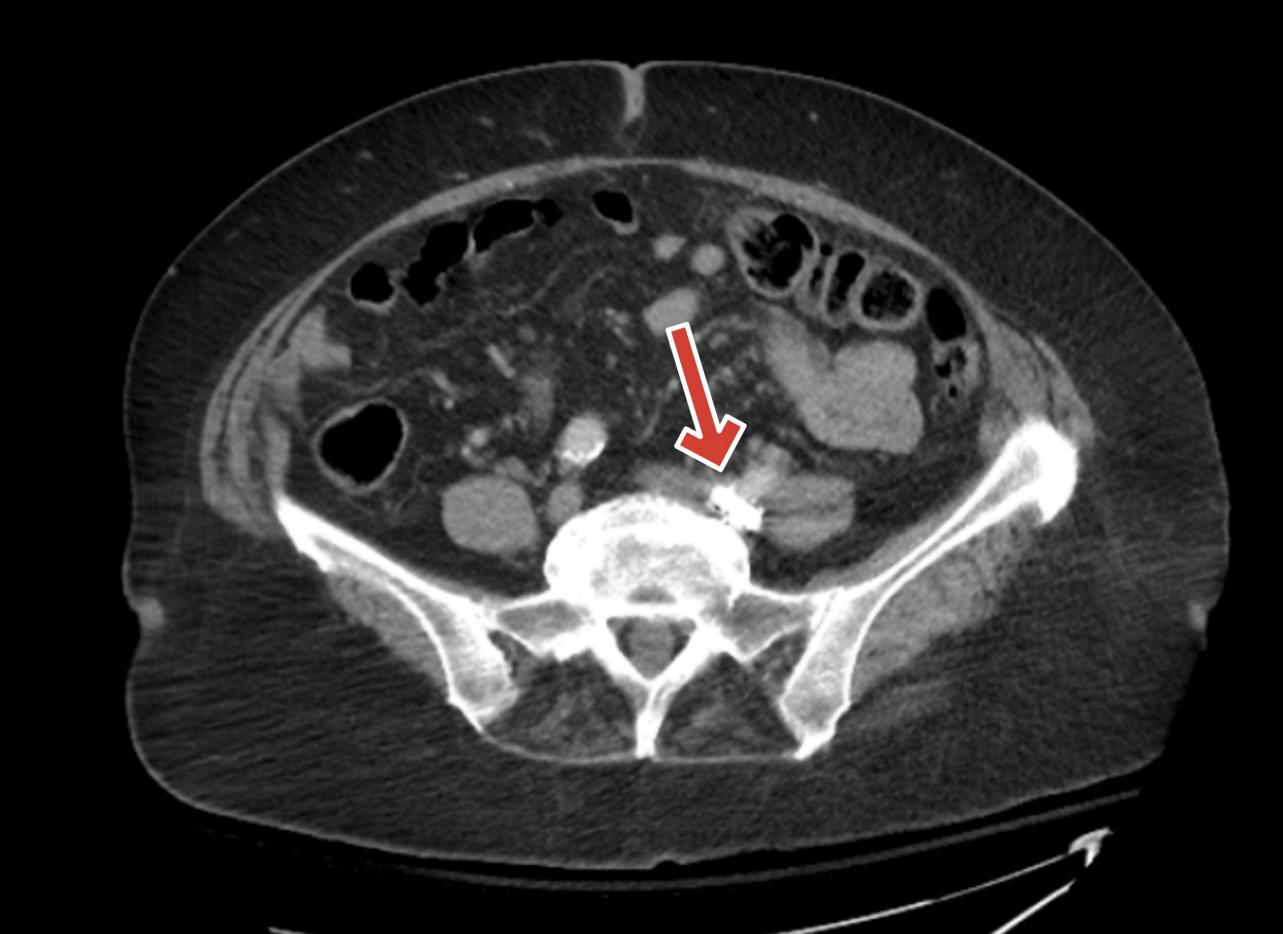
CT of the abdomen/pelvis with IV contrast with 3-cm radio-opaque density (arrow points at the foreign radio-opaque object) CT: computed tomography
